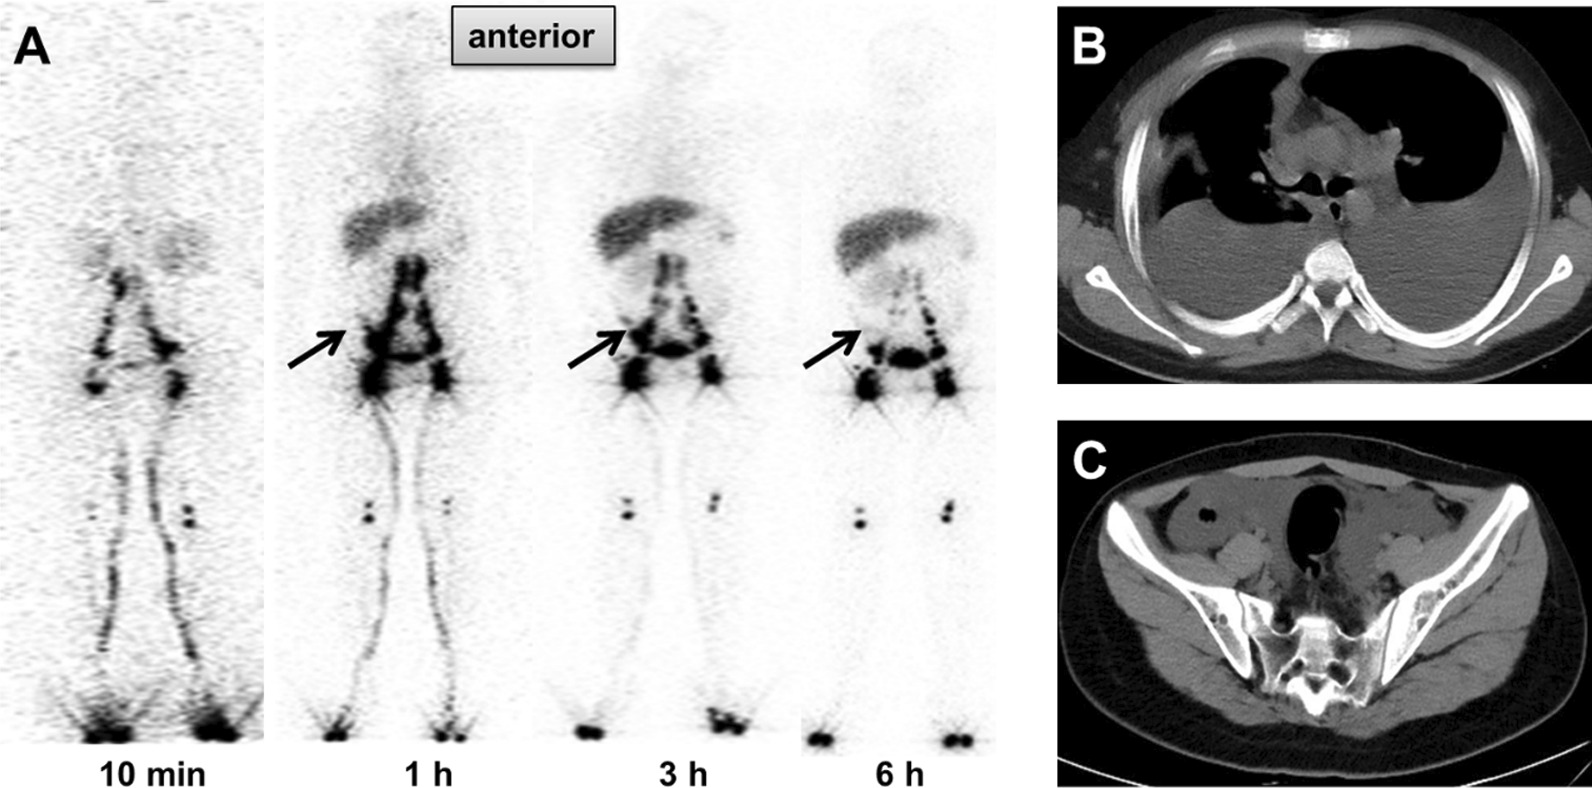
A 14-year-old male with bilateral pleural effusion and ascites. The right-sided pleural effusion confirmed exudative chylothorax. A The anterior lymphoscintigraphy images acquired at 10 min–6 h showed no elevated radioactivity in the bilateral thoracic cavities and arc-shaped abnormally elevated radioactivity in the pelvic cavity (black arrows). B, C The CT aerial views of the chest and pelvis showed massive liquid in the bilateral thoracic cavities and abdominal cavity
